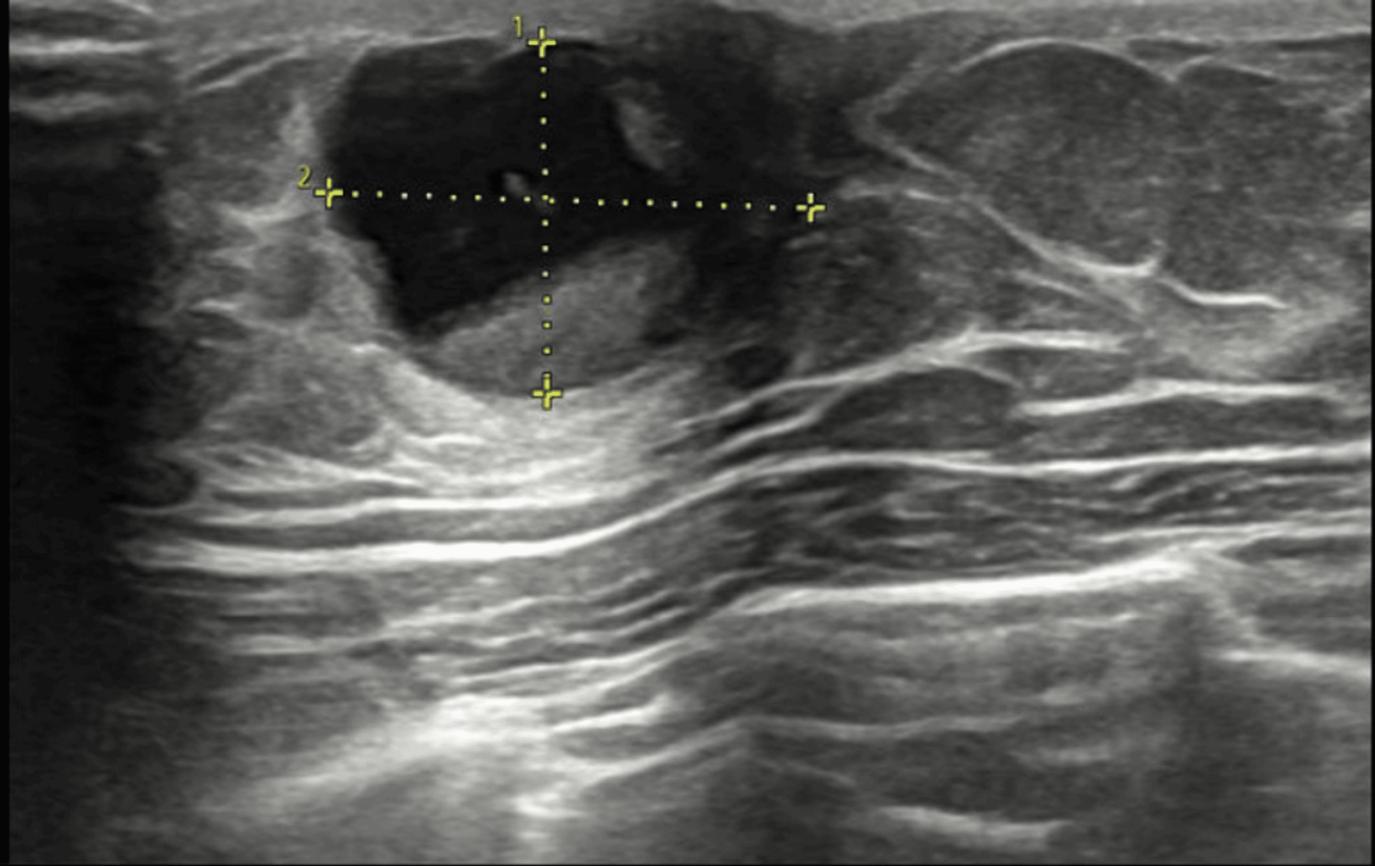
Ultrasonography breast done as a part of the triple assessment The well-defined oval-shaped mixed solid and cystic lesion measured 15 x 13 mm and was located at the 12 o'clock position of the left breast. The lesion shows a solid component adherent to the wall measuring approximately 10x11mm. Imaging features were consistent with a suspicious breast lesion. No other lesions in the left breast were seen. Suspicious-appearing lymph nodes were noted in the left axilla with maintained fatty hilum.
